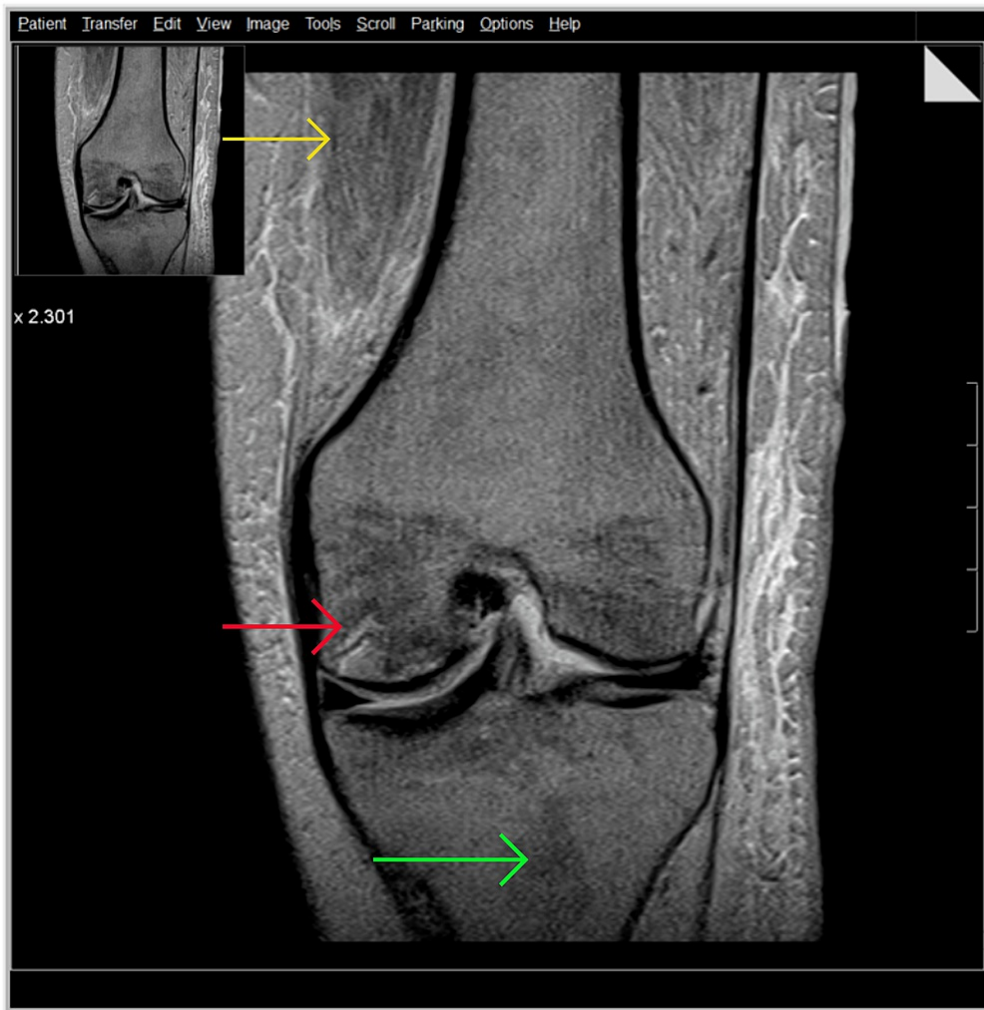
Knee MRI coronal view Yellow arrow identifies atrophic musculature; red arrow highlights areas of alternating hyper and hypointensity and serpiginous lines within the subchondral marrow of the medial and lateral femoral condyles; green arrow shows bone marrow heterogeneity.
